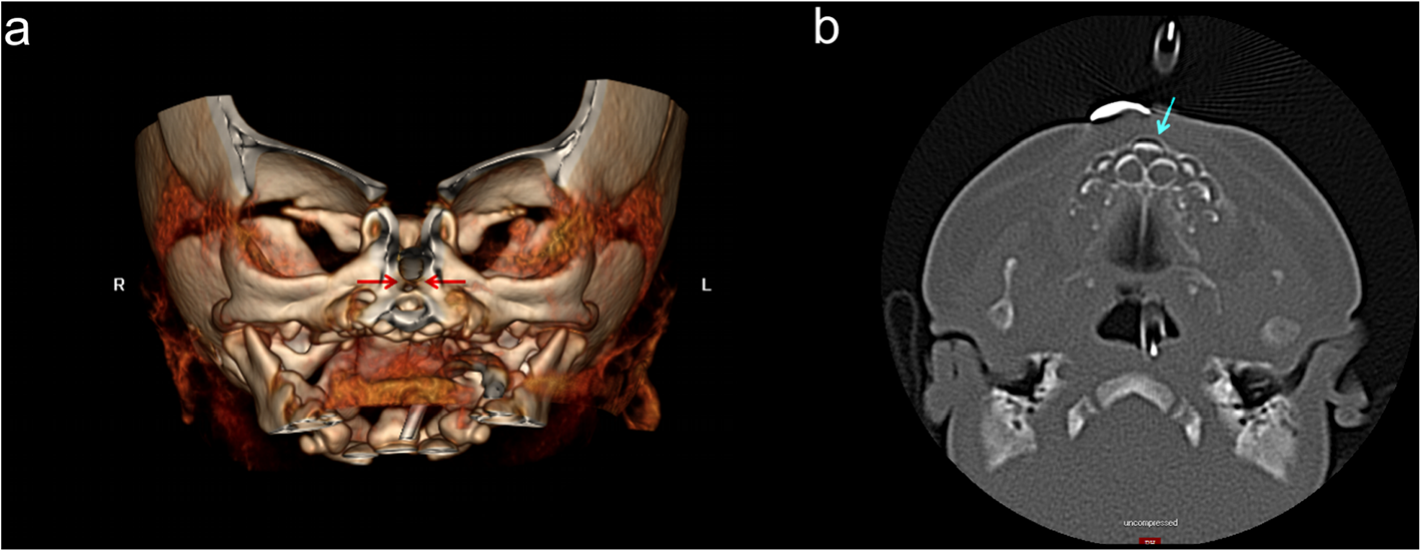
Computed tomography scans showing congenital nasal pyriform aperture stenosis and solitary median maxillary central incisor. A three-dimensional reconstructed computed tomography scan shows the amount of congenital nasal pyriform aperture stenosis (arrows). R, right; L, left. A maximum diameter of only 4.9mm is apparent (a). A computed tomography scan reveals a solitary median maxillary central incisor (arrow) precisely in the midline of the maxillary dental arch (b).
